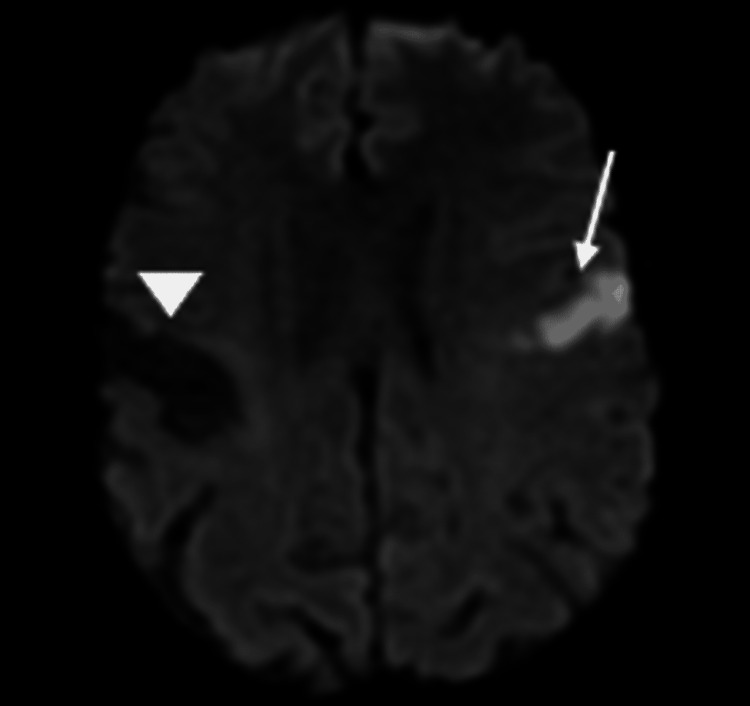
Diffusion-weighted MRI of the brain showing increased signal intensity in the region of the left frontal operculum (arrow), which indicates an acute stroke. Decreased signal intensity in the region of the right frontal operculum (arrowhead) is from a chronic infarct.
